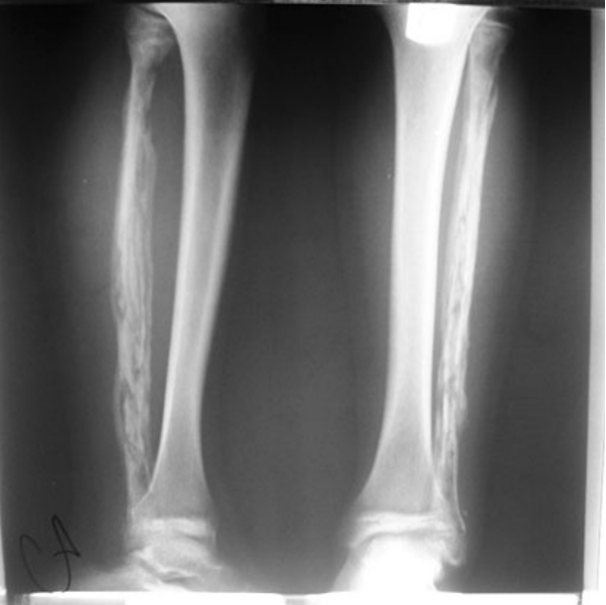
Radiograph showing mixed density, periosteal reaction, and cortical disruption with soft tissue swelling in metaphysis of left fibula
